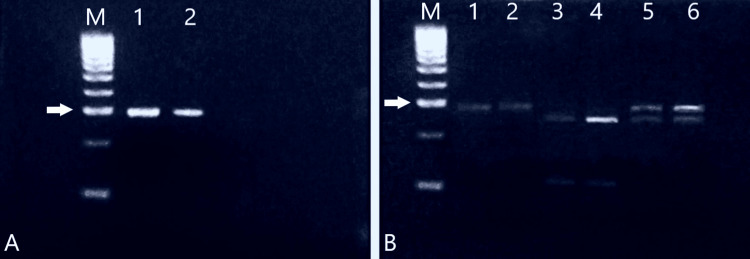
PCR results (A) M–DNA marker across 100 nucleotides (nt), 1 and 2 are samples after PCR and gel purification. (B) Result after restriction analysis: M–DNA marker through 100 nt, 1 and 2 - fragments not cut by the enzyme, homozygous TT, 3 and 4 - homozygous CC, 5 and 6 - heterozygous CT genotype. White arrow – 300 nt
